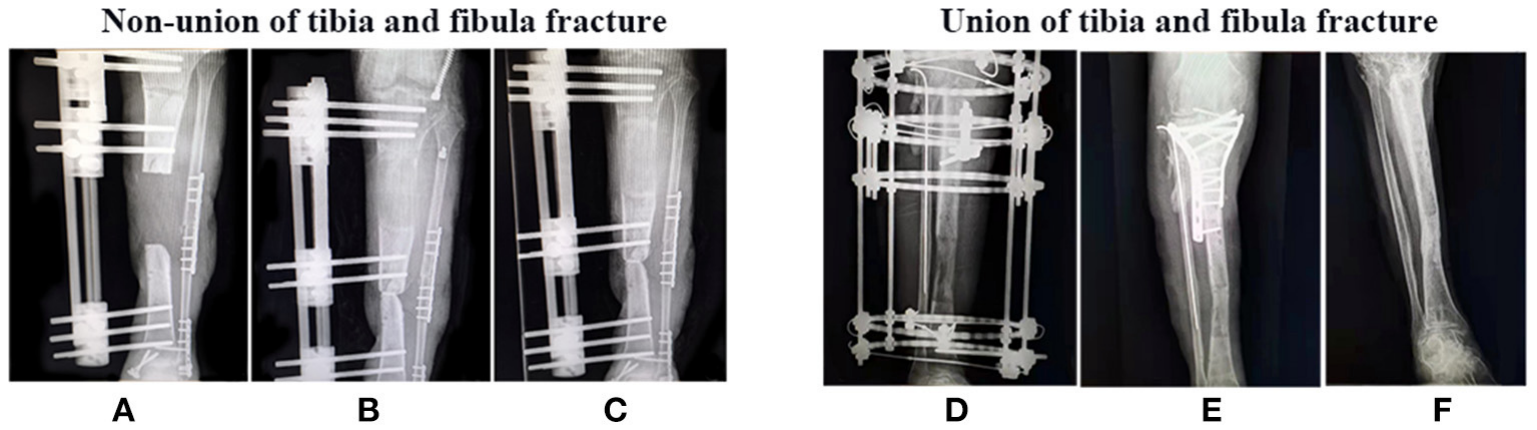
Non-union and Union of tibia and fibula fracture after surgery. X-ray examination (A,D) 1 month, (B,E) 3 month, and (C,F) 9 month after surgery.
